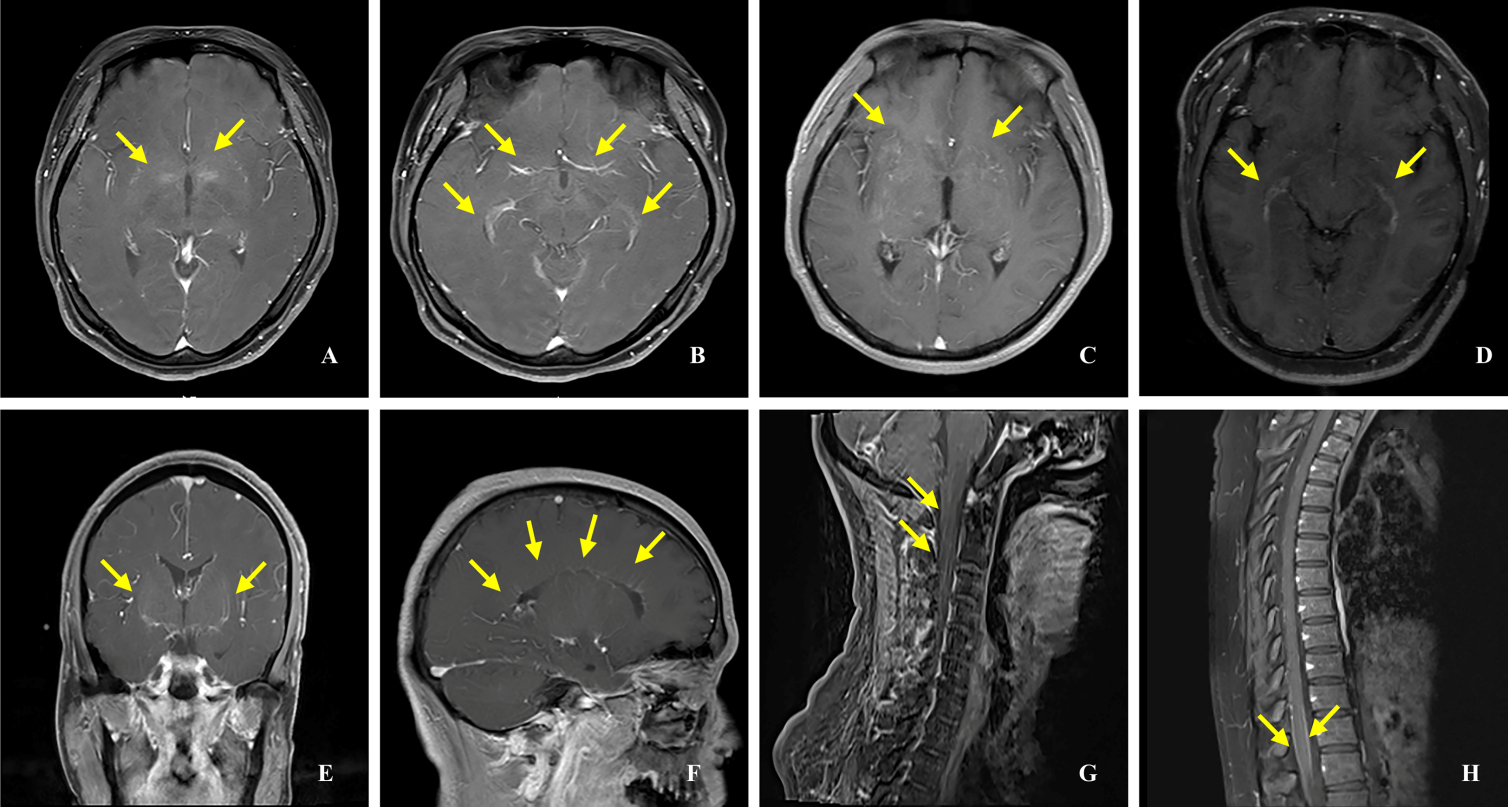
Gadolinium enhancement MRI in patients with anti-GFAP antibodies. (A, C) Punctate enhancement lesions. (B, D) Periependymal enhancement. (E) Linear enhancement of the cerebellum. (F) Linear enhancement perpendicular to the ventricle. (G) Linear enhancement of the spinal cord. (H)Enhancement of the spinal cord membranes.
